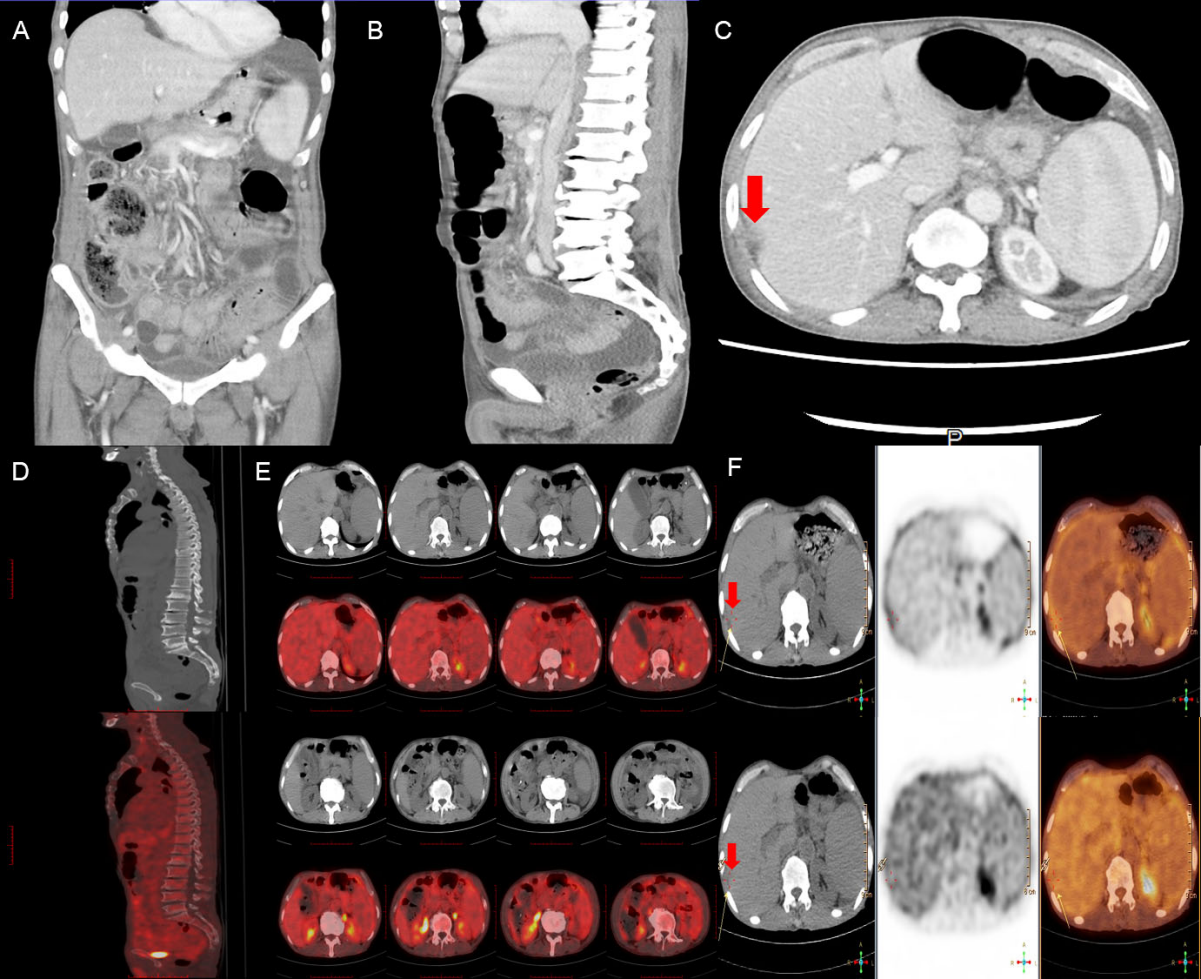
Contrast-enhanced abdominal CT (A-C) scan showed volumetric disproportion of lobar and segmental of the slightly enlarged liver with massive abdominal-pelvic fluid accumulation. Full-body PET-CT scan (D-F) showed no significant hypermetabolism but a slightly low signal from the subcapsular area in the right hepatic lobe with ascites and splenomegaly. Red arrows point to the subcapsular area in the right hepatic lobe with ascites.
